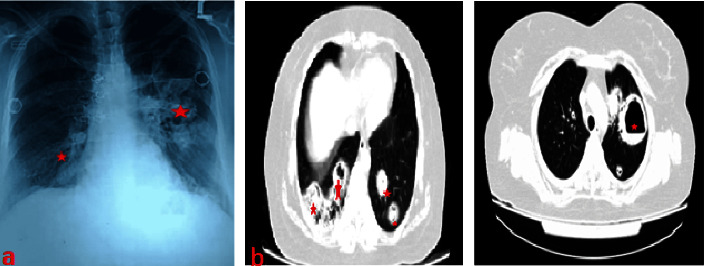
(a) X-ray chest (b) high-resolution computerized tomography (HRCT).
